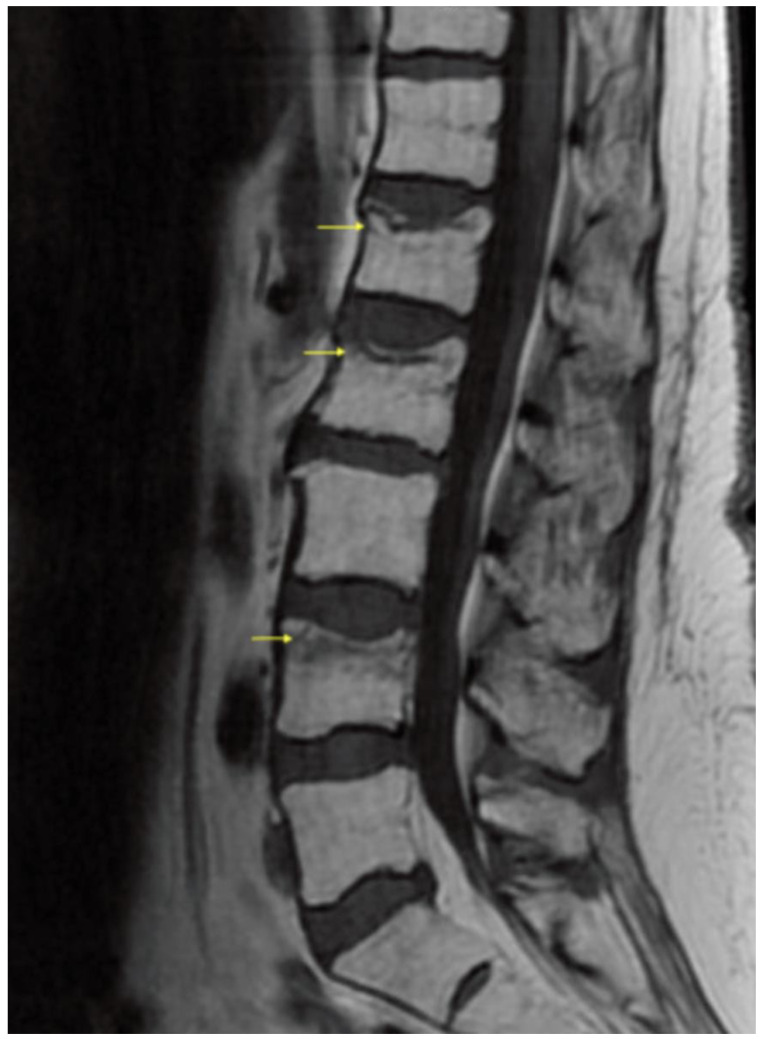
Lumbar spine magnetic resonance T1 image (MRI) with yellow arrows demonstrating superior endplate vertebral compression fractures of the L1, L2 and L4. In particular, the prominent bone marrow edema of the L4 vertebral endplate is consistent with an acute/subacute compression fracture.
